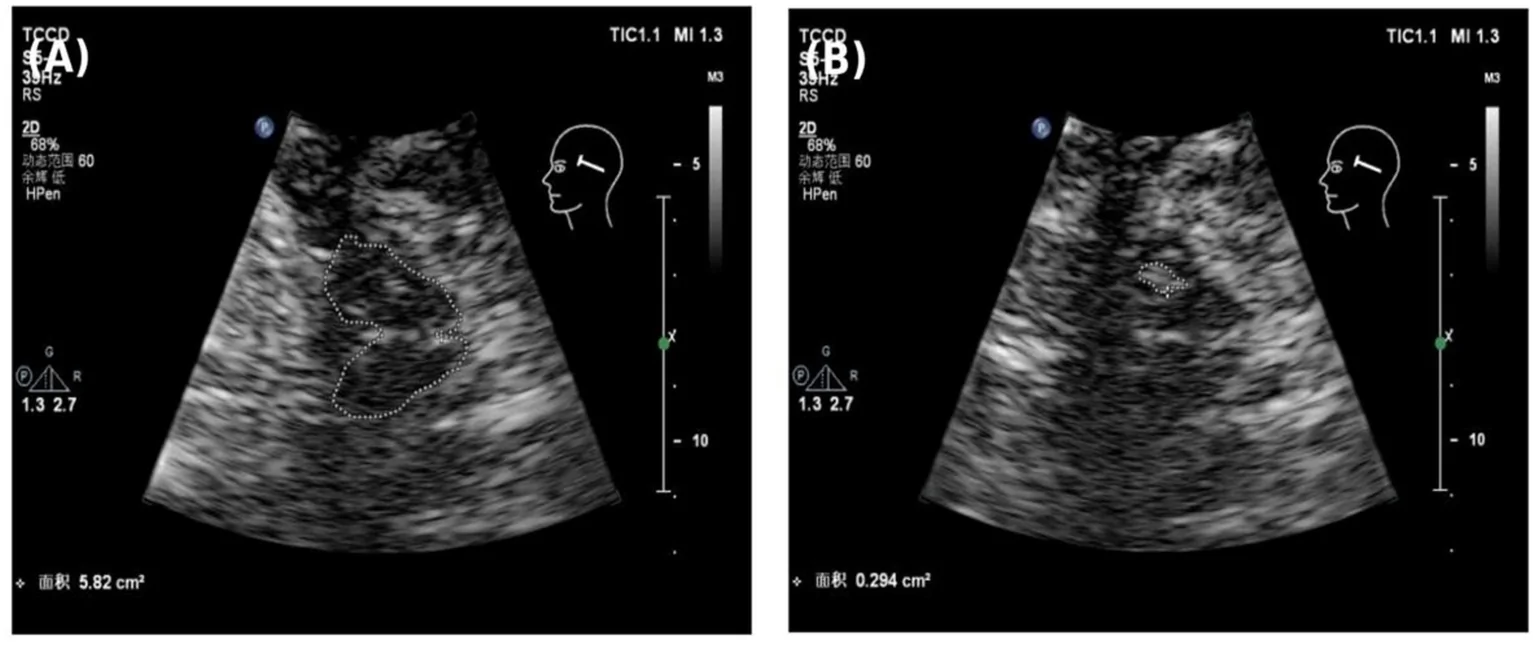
Transcranial sonography of the midbrain and substantia nigra in a PD patient. (A) Midbrain area measurement (total area = 5.82 cm^2^); (B) substantia nigra hyperechogenicity area measurement (area = 0.294 cm^2^).
